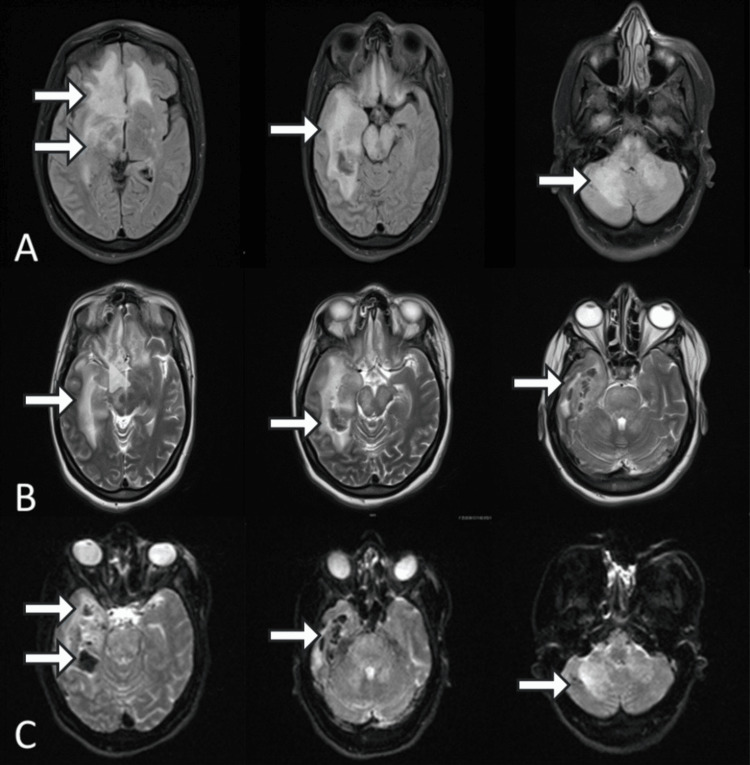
Brain MRI at admission (A) Axial FLAIR-weighted images demonstrate multiple bilateral cortical-subcortical hyperintense lesions predominantly involving the frontal lobes and insular cortices, with extension to the temporal lobes, associated with surrounding vasogenic edema (white arrows). (B) Axial T2-weighted images confirm extensive hyperintense lesions with heterogeneous signal intensity, suggesting areas of necrosis in the frontal, insular, and temporal regions (white arrows). (C) Susceptibility-weighted imaging (SWI)/T2-weighted images reveal multiple punctate hypointense foci within the lesions, consistent with microhemorrhages (white arrows).
